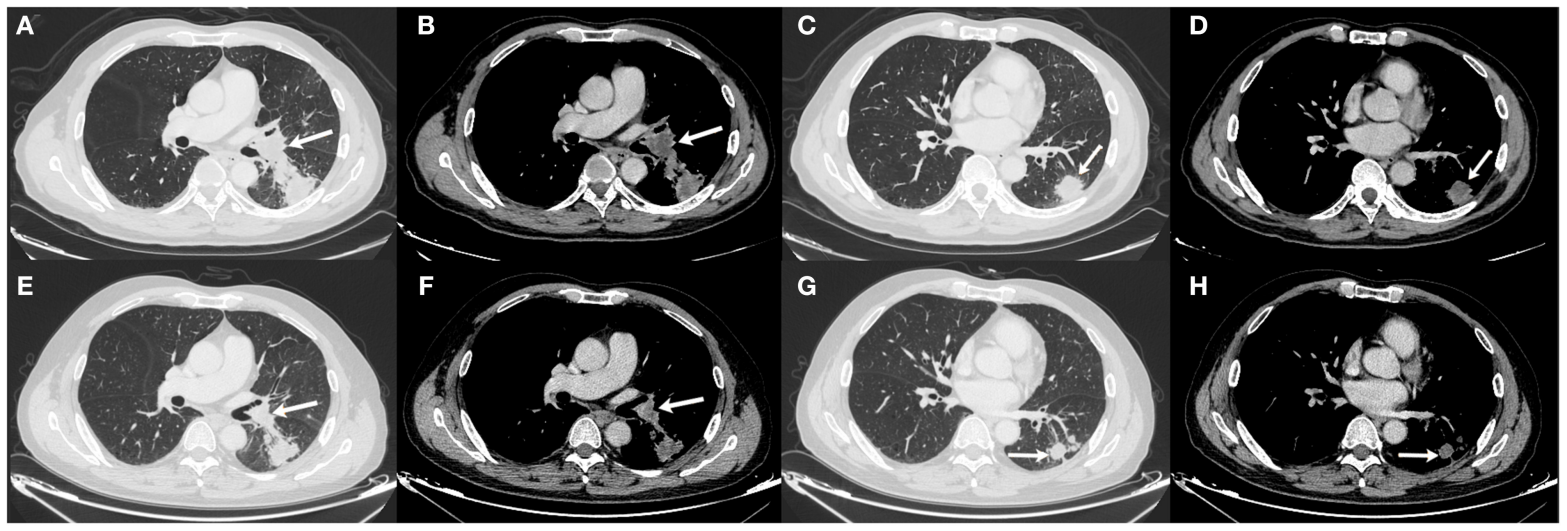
Chest CT scan of the masses on December 6th, 2019 (A–D) and March 15th, 2020 (E–H). (A,B) The size of the mass adjacent to the hilum was 2.2 cm × 3.3 cm × 2.1 cm on December 6th, 2019; (C,D) The size of the mass on the basal segment was 2.9 cm × 3.0 cm × 2.1 cm on December 6th, 2019; (E,F) The size of the mass adjacent to the hilum was 2.1 cm × 2.5 cm × 1.9 cm on March 15th, 2020; (G,H) The size of the mass on the basal segment was 2.0 cm × 1.9 cm × 1.8 cm on March 15th, 2020. White arrows indicate the locations of the masses.
